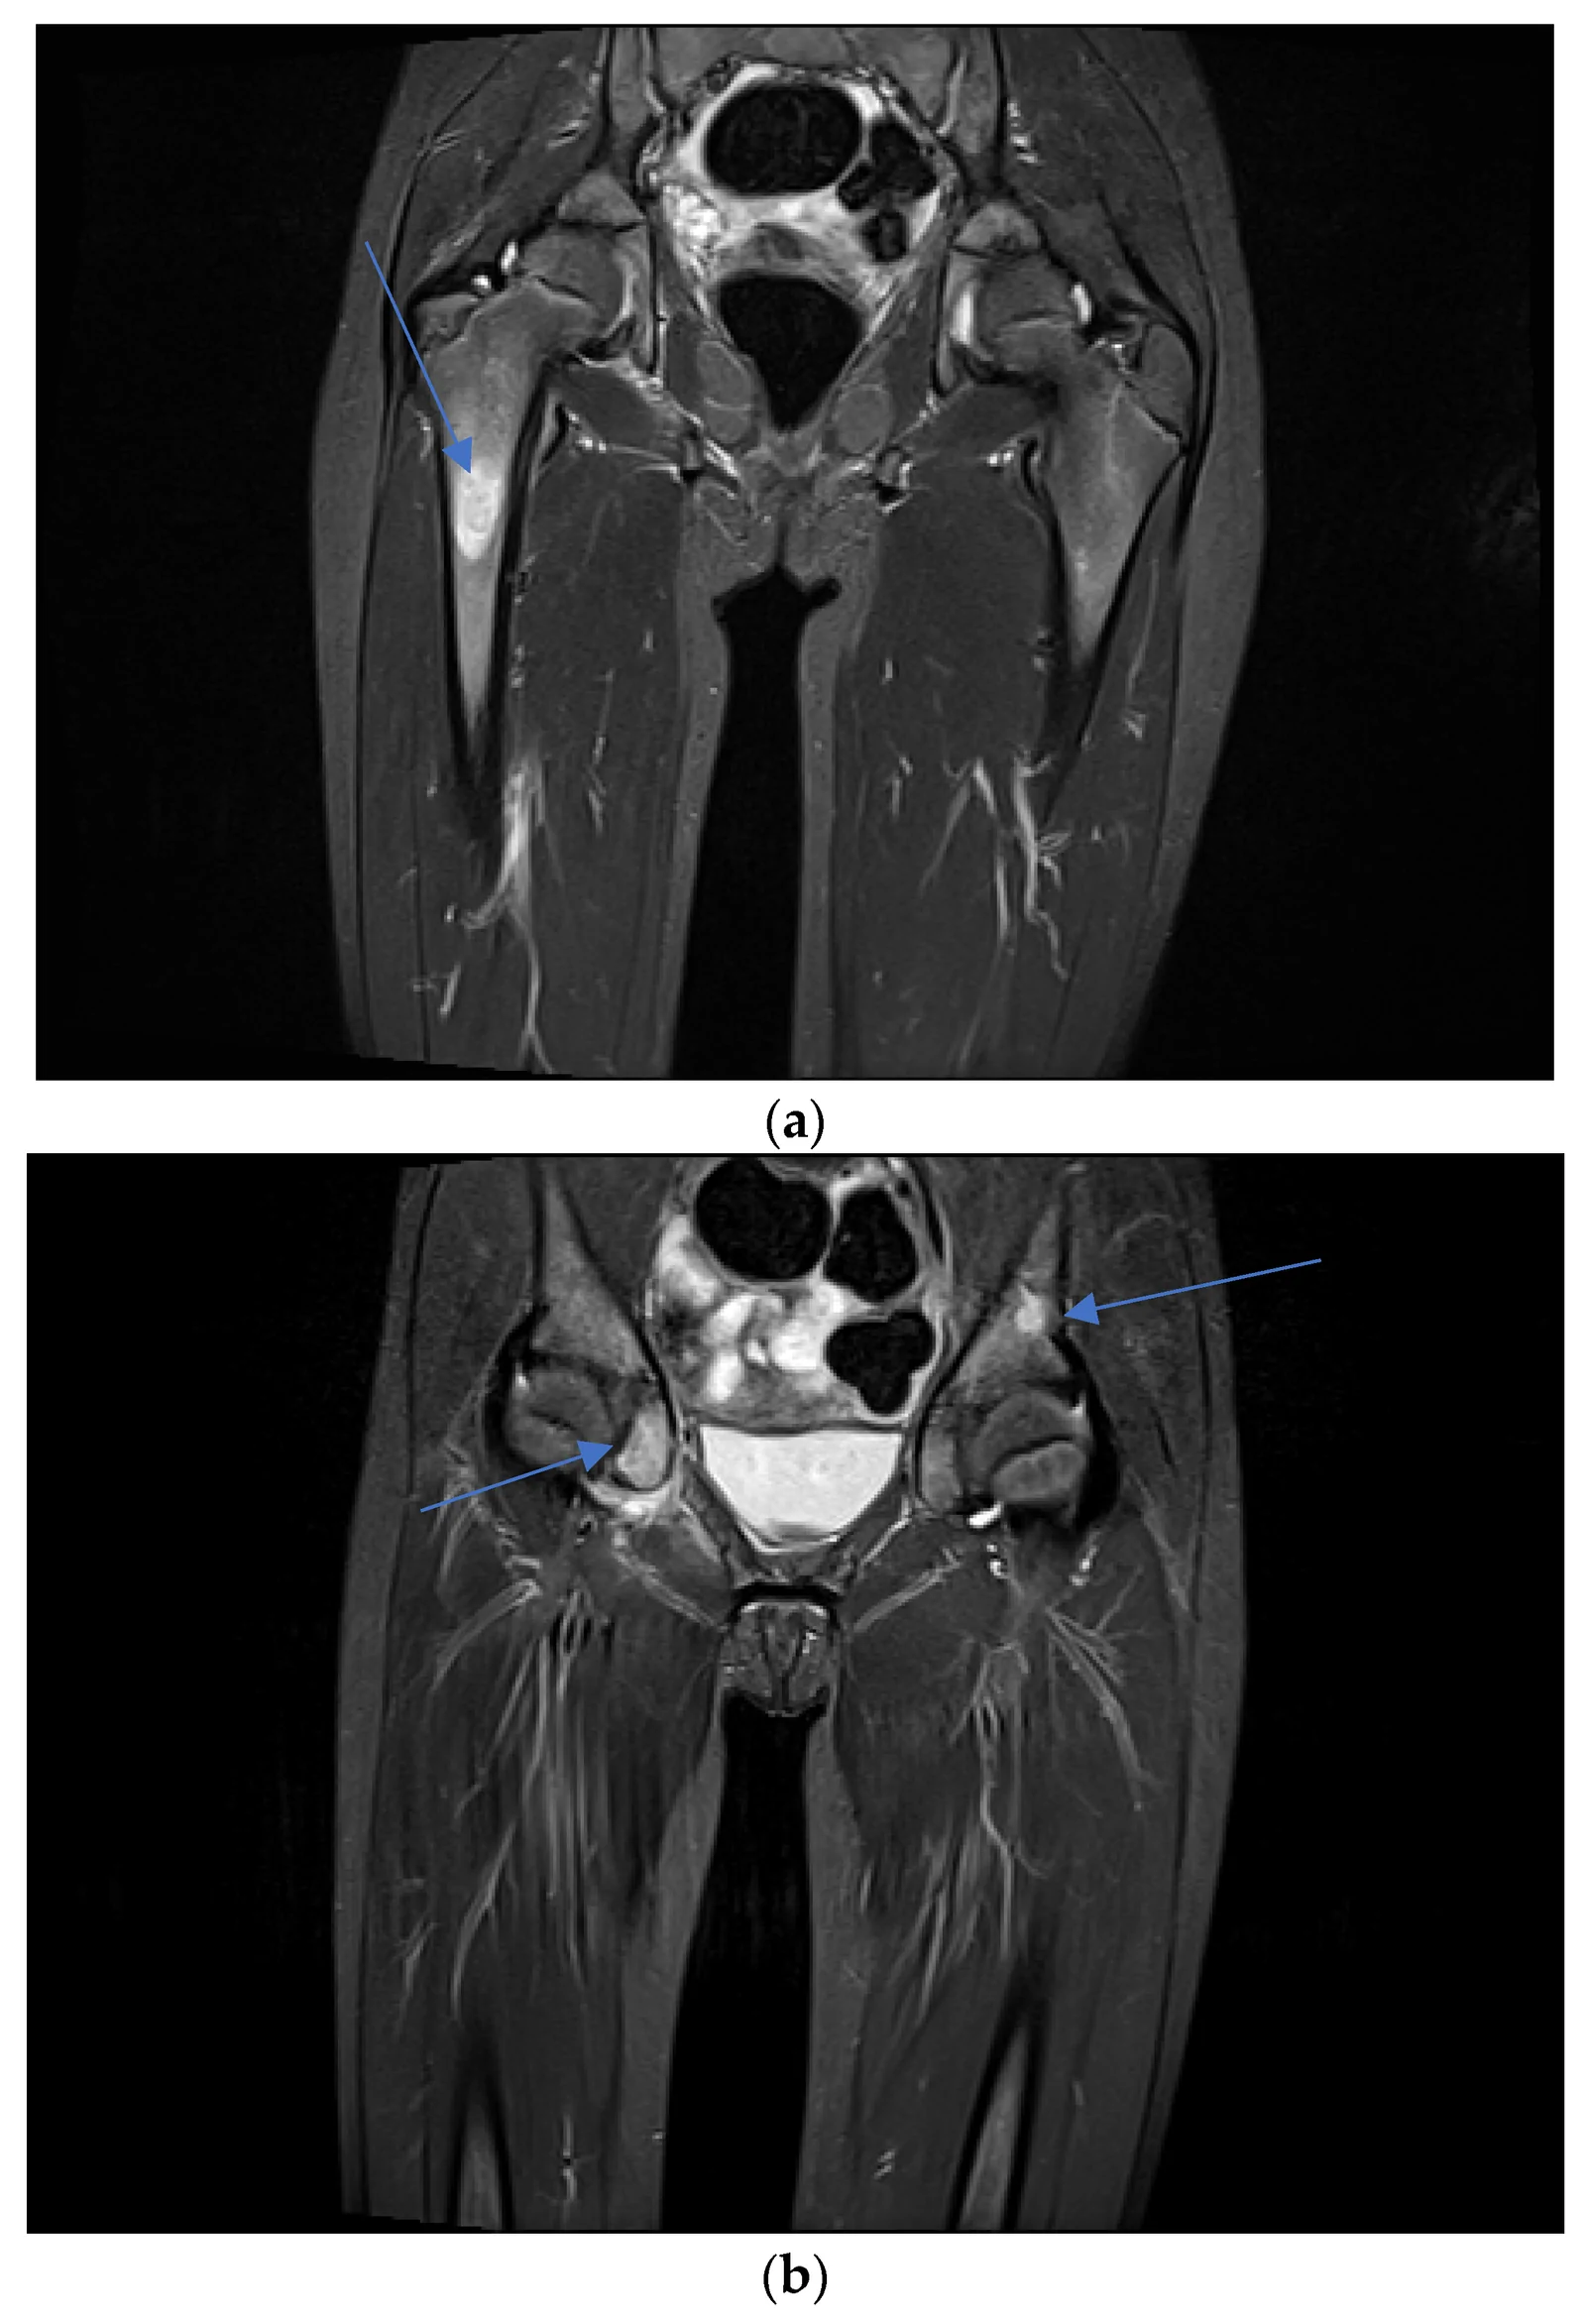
(a). Coronal T2-weighted MRI image showing a lesion of the right femur (arrow). (b). Coronal T2-weighted MRI image showing lesions of the right pubic bone and left iliac wing (arrows).
